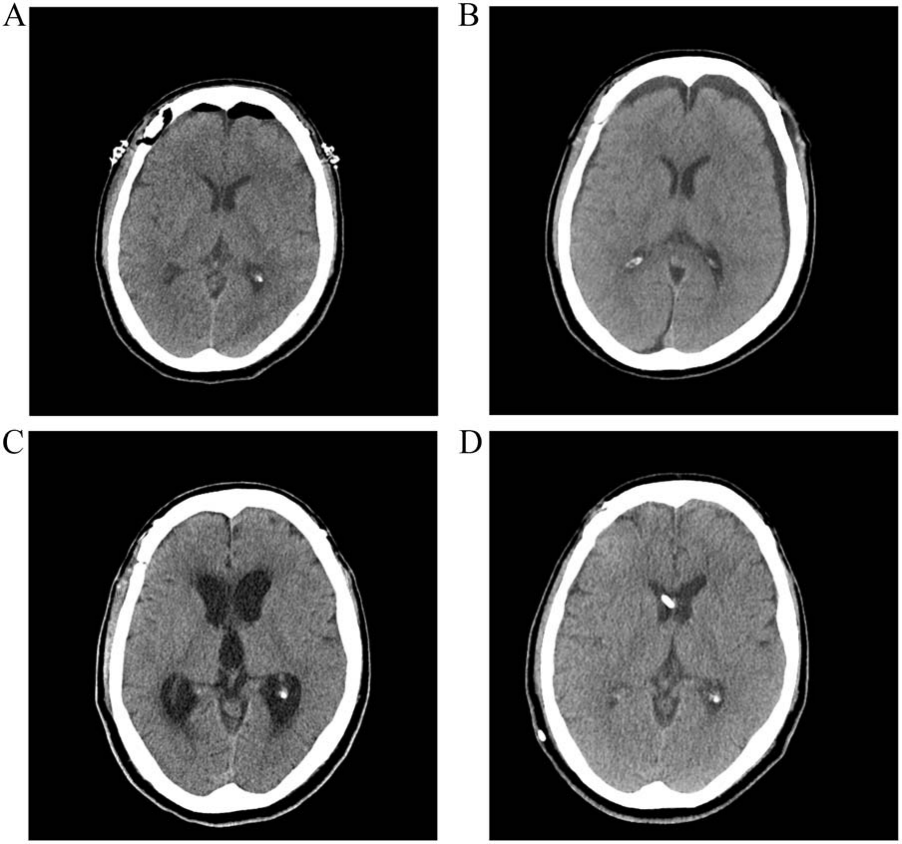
(A) Postoperative CT scan showing no subarachnoid or intraventricular hemorrhages. (B) Bilateral subdural fluid collection on postoperative day 13 CT scan. (C) Increased ventricle size by day 26. The patients developed symptoms of hydrocephalus. (D) A ventriculoperitoneal shunt was placed on day 47. Ventricle size decreased and clinical signs of hydrocephalus improved.
